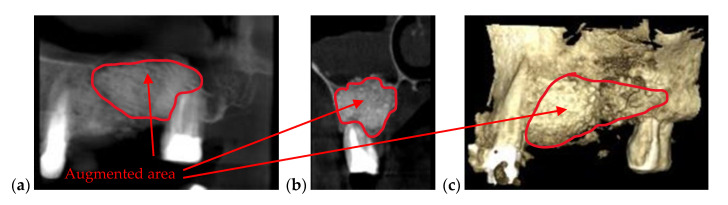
(a) Panoramic view, (b) sagittal view, and (c) 3D reconstruction of an augmented bone obtained after a segmental 3D CBCT with a FOV of 5 × 5 cm. Patient P.P., male, age 46 years, diagnosed with severe periodontitis.
